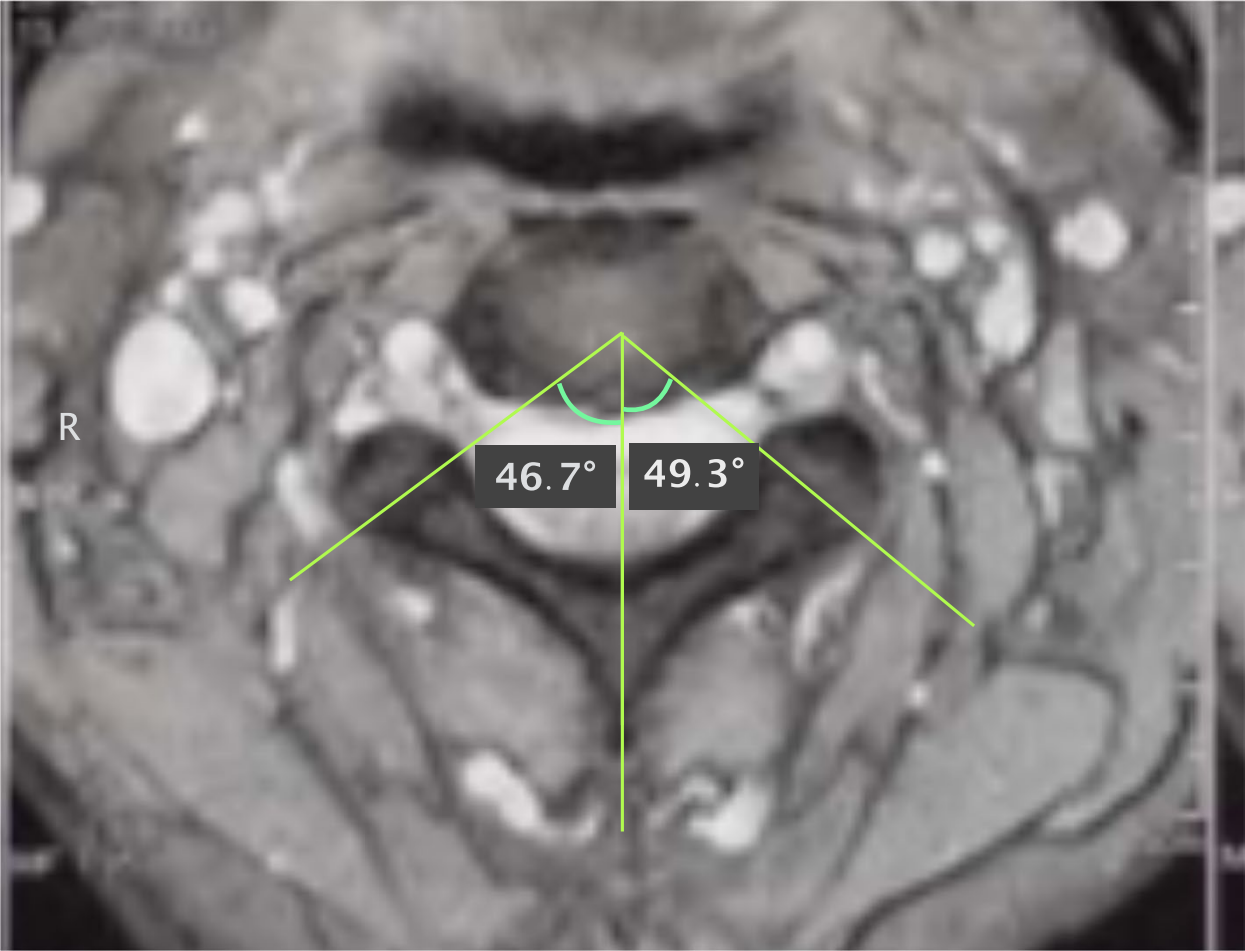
Measurement method of the facet joint angle and anteroposterior diameter (A-P diameter) of spinal canal in the transverse MRI image. A facet line was drawn connecting the anterior and posterior margins of each of the superior articular facet. Then, a mid-sagittal line was added that passed through the center of the base of the spinous process. The angle between the facet line and the midsagittal line was defined as the facet angle
